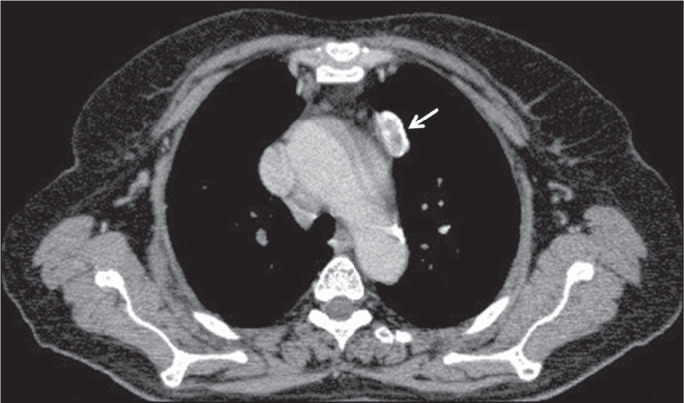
A 71-year-old female with a hydatid cyst of unknown origin. Chest CT
